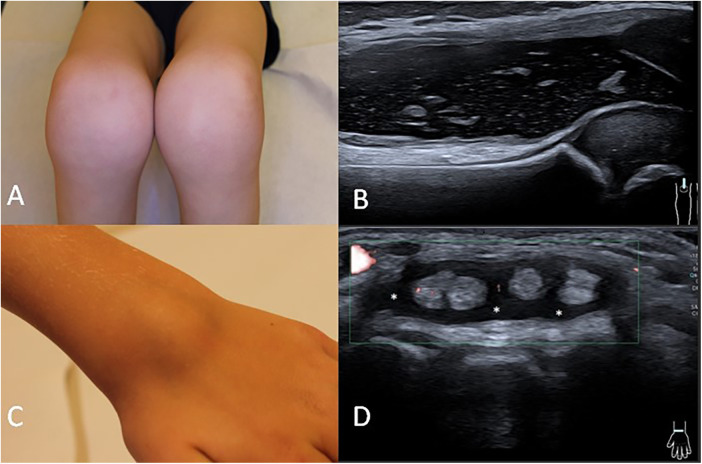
The panel shows clinical and ultrasound images from two patients with CACP. Panels A and C demostrate clinical features of symmetrical knee joint swelling and swelling of the wrist extensor tendon region, respectively. Panels B and D show the corresponding ultrasound images. Panel B demonstrates a large effusion (BM grade 3) with hyperechoic foci within an otherwise anechoic effusion and absence of synovial hypertrophy in the suprapatellar longitudinal view. Panel D shows an anechoic effusion of the extensor tendon sheath in the transverse view. BM, B-mode; CACP, Camptodactyly-arthropathy-coxa vara-pericarditis syndrome; CI, confidence interval; JIA, juvenile idiopathic arthritis.
